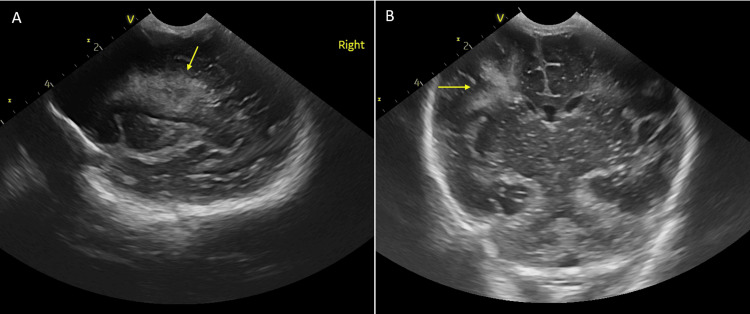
Cranial ultrasound imaging of twin A, performed via the anterior fontanelle on the second day of life A: Parasagittal view demonstrating periventricular hyperechogenicity localized to the right frontoparietal region (arrow).
